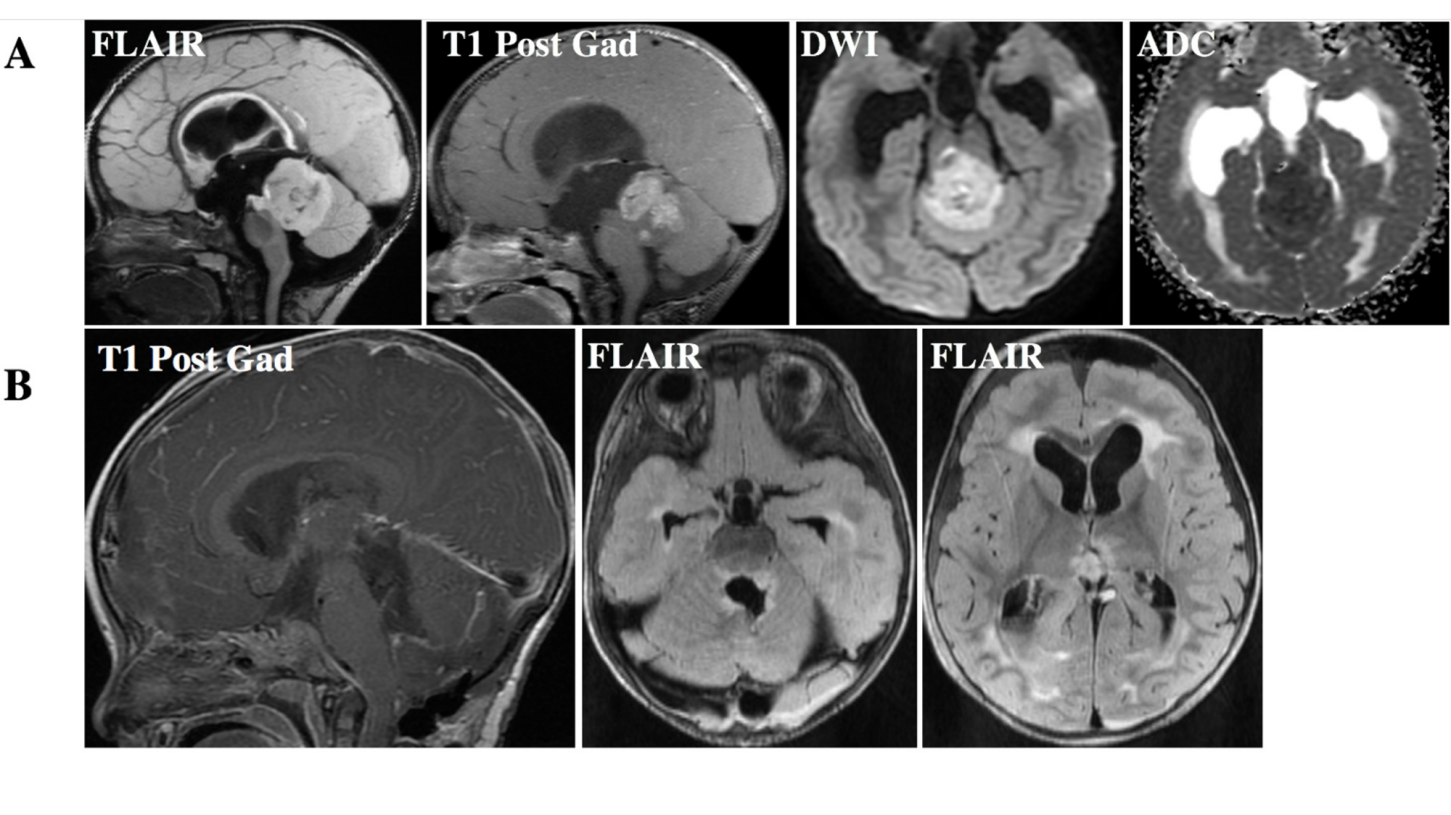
Pineoblastoma with the DICER mutation at presentation and following resection A. FLAIR hyperintense and enhancing lesion involving the pineal gland with inferior displacement of the vermis. The lesion demonstrates prominent reduced diffusion, indicating high cellularity. B. Evidence of gross total resection of the tumor on post-gadolinium imaging. FLAIR imaging demonstrates post-surgical changes within the resection bed and evidence of an intraventricular hemorrhage. FLAIR: fluid attenuated inversion recovery
